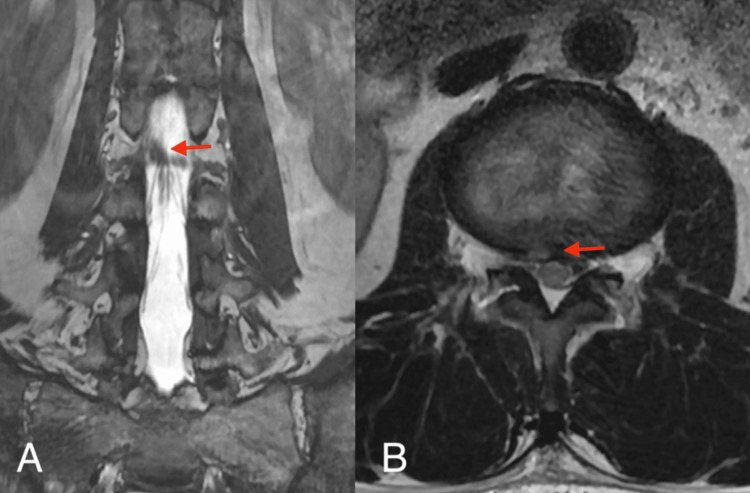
Three-month postoperative MRI (A) Although the position of the exiting nerve root remained unchanged in the coronal MRI, a reduction of upward herniation was observed (arrow). (B) The axial MRI showed the reduction of the herniation that was protruding from the spinal cord (arrow).
